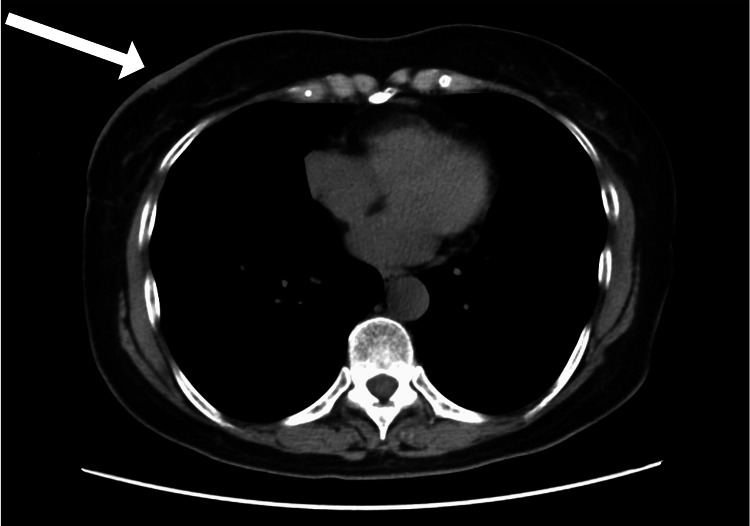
Chest computed tomography (CT) findings The section showed thickening of the skin in the right breast, but no evident mass lesion was found in the mammary gland.
